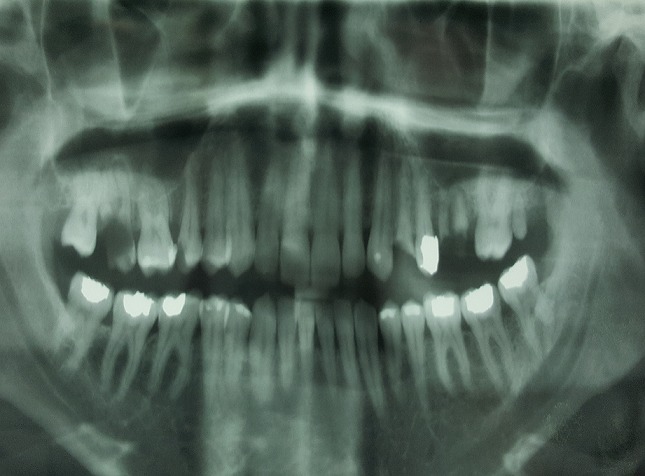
Orthopantomogram of the patient
